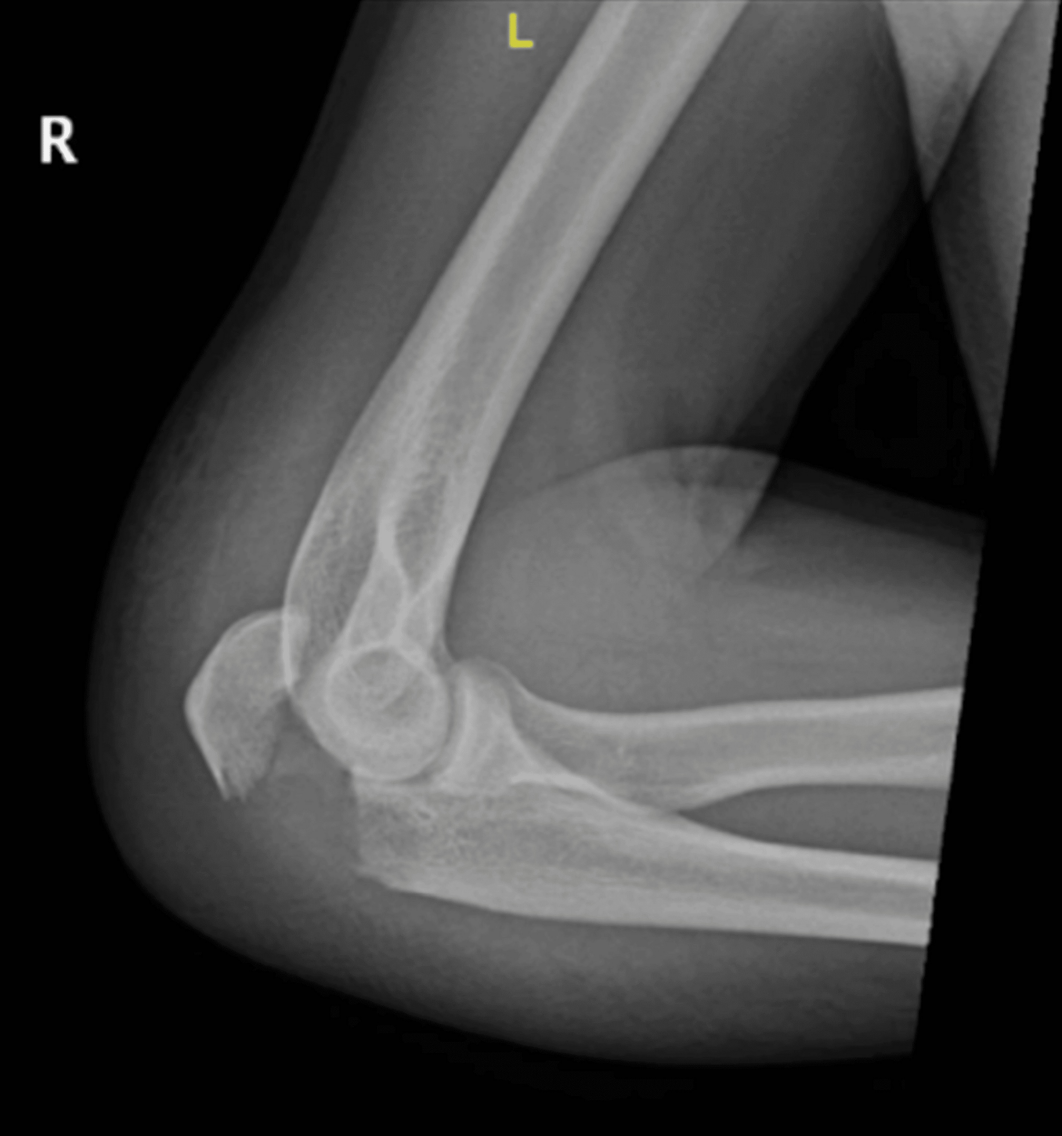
Preoperative lateral X-ray image of the right elbow.
